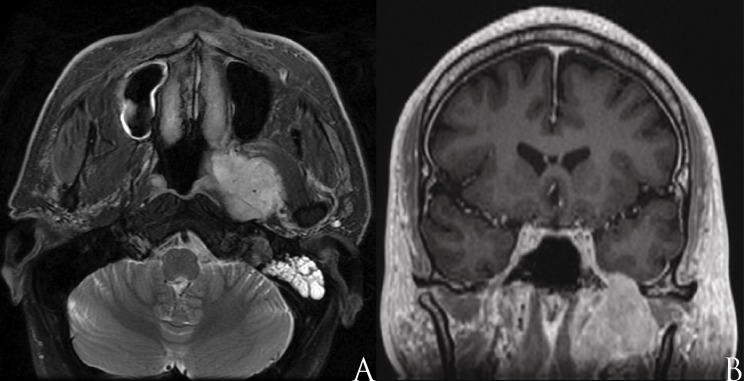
Preoperative magnetic resonance imaging. Axial T2-weighted magnetic resonance (MR) with gadolinium contrast reveals a mass located in the left parapharyngeal space (A). The lesion invades the pterygopalatine fossa and displaces the lateral pterygoid muscle anteriorly. (B) Coronal T1 post-gadolinium MR reveals the mass to extend to the intracranial space through foramen ovale without intradural or cavernous sinus invasion.
